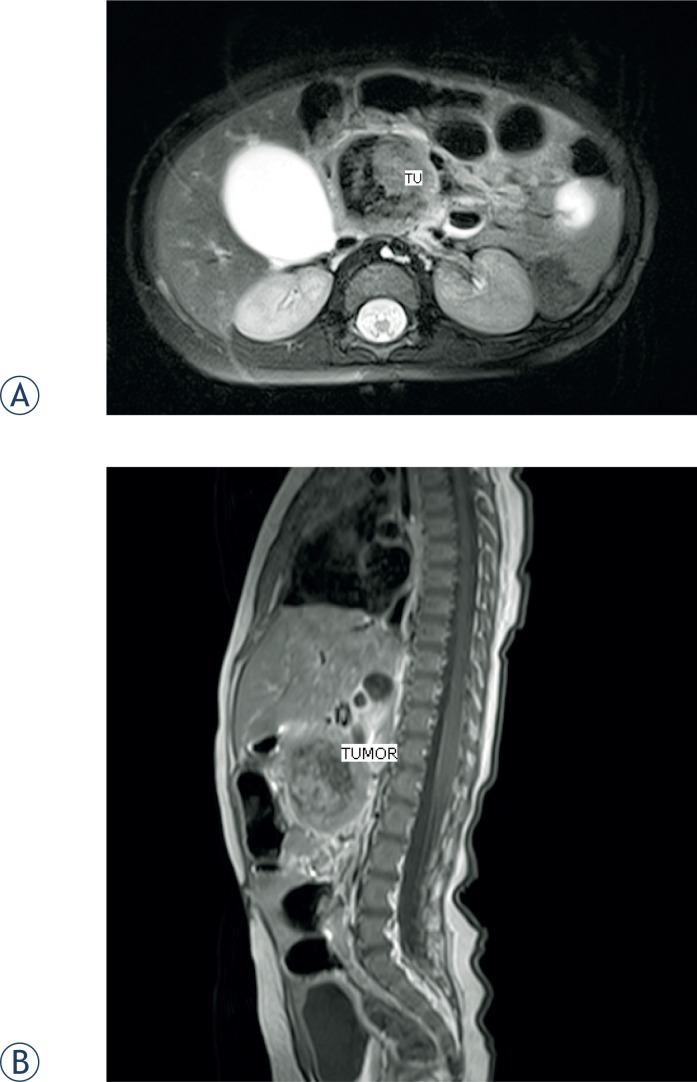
MRI confirmed well circumscribed tumor mass, with a diameter of 37 mm. The origin of the mass was in the head of the pancreas and in the uncinate process. There was no infiltration of the surrounding tissue. Tumor impressed the caval vein and pushed the superior mesenteric artery and vein ventraly and lateraly. (A) Coronal plane. (B) Sagital plane.
